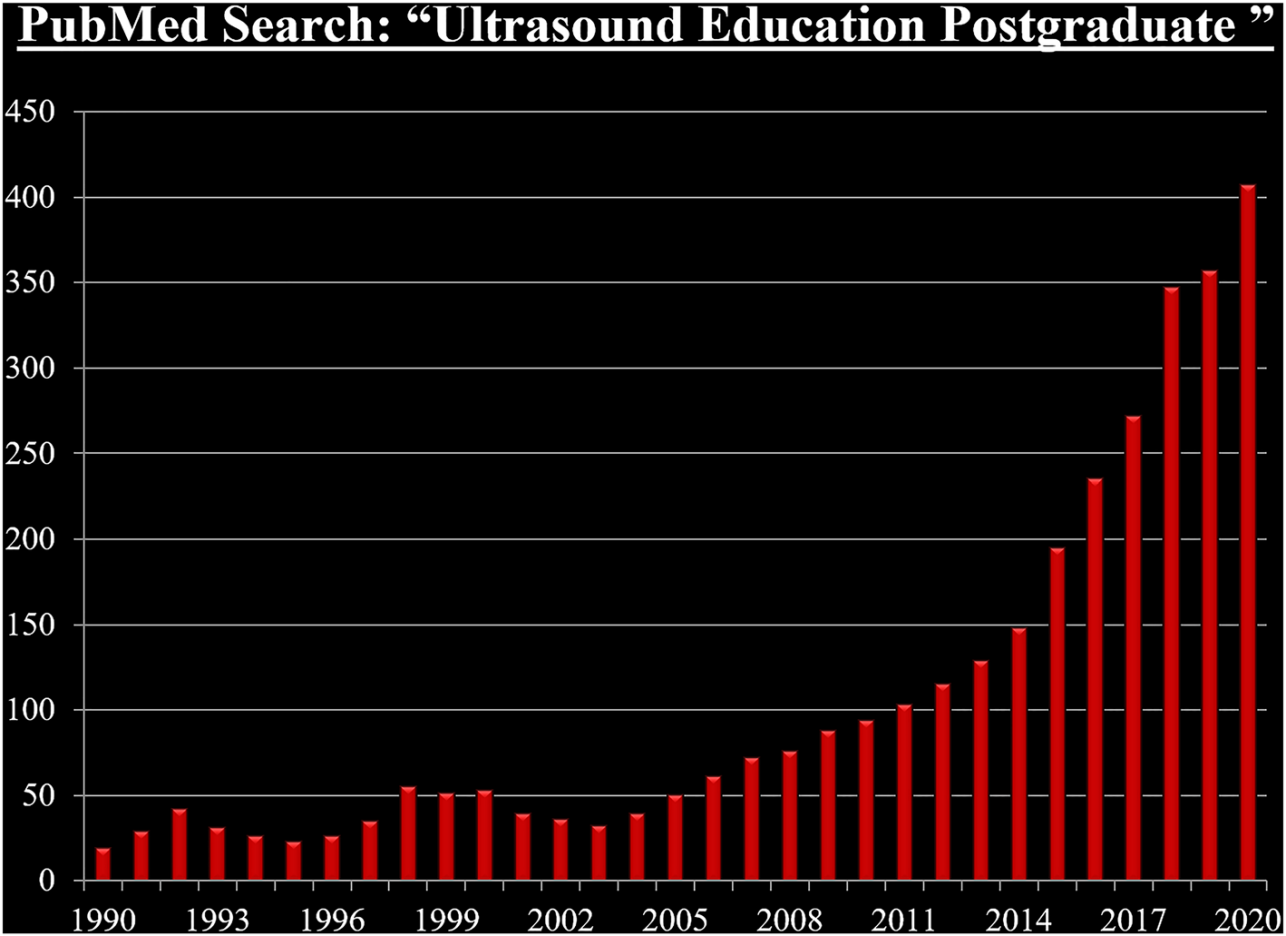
A PubMed search of articles using *ultrasound education postgraduate* as the query
